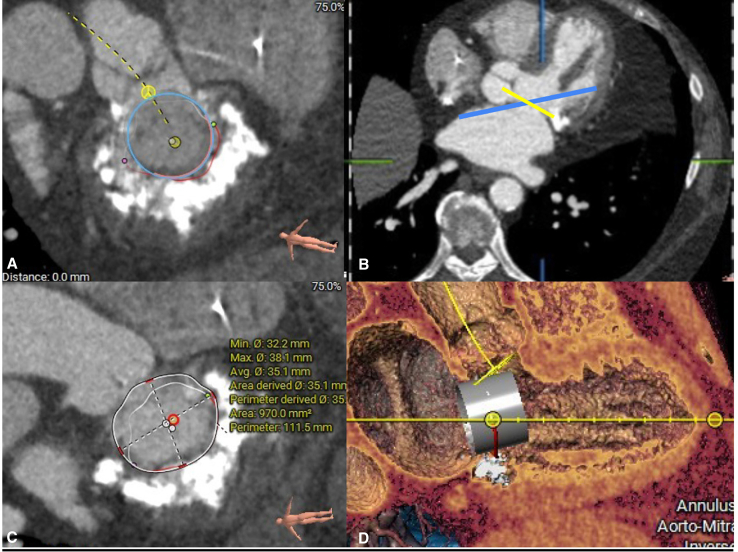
Computed tomography angiography (CTA) recon with mitral annular calcification (MAC) details and angle recon for case 1. A, Multiplanar reformatting (MPR) demonstrating severe MAC. The *yellow dotted line* represents deployment angle and distance. B, Axial multiplanar reformation MPR, mitral annulus (*yellow line*) with direction of nose cone on deployment system (*blue line*). C, MPR with planned landing zone (annulus area, 7.3 cm2; aorta-mitral angle, 63.4°; inverse angle, 116.6°). D, Volume rendering of double-oblique MPR demonstrating valve positioning. The *yellow dotted line* represents deployment angle and distance.
